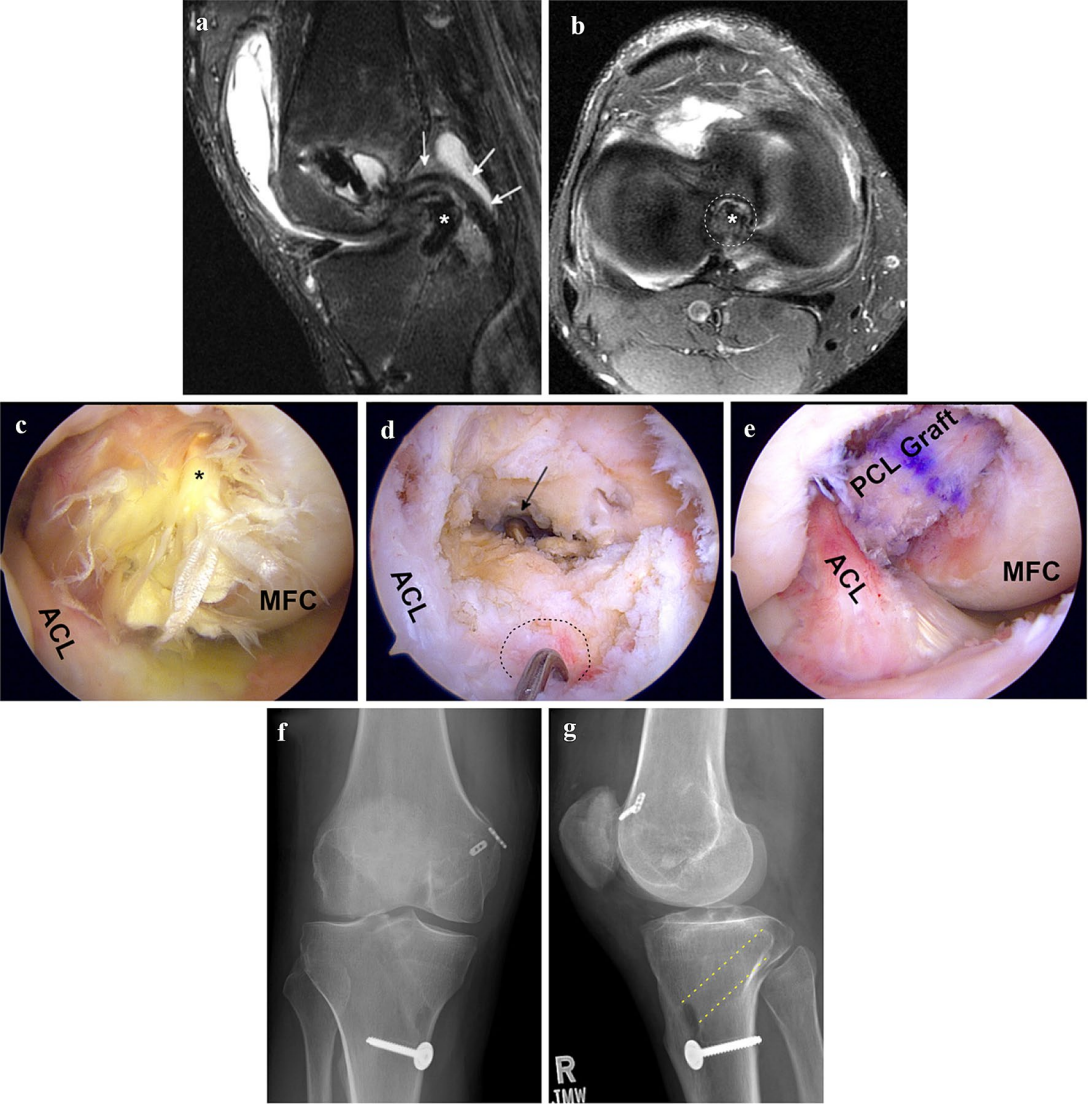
Posterior cruciate ligament graft failure. Patient with atraumatic PCL graft failure of the right knee. T2-weighted sagittal (a) and axial (b) MR images showing PCL graft failure and misplaced tibial tunnel (too anterior and too proximal). Note scarring of remnant PCL fibers imitating PCL continuity (white arrows). Arthroscopic images demonstrating graft failure (c), misplaced tibial tunnel (d), and revision PCL graft (e). Postoperative anterior–posterior (f) and lateral (g) radiographs demonstrating new anatomic tibial tunnel. white/black dashed lines, misplaced tibial tunnel; yellow dashed lines, new anatomic tibial tunnel; black arrow, new anatomic tibial tunnel during revision PCL reconstruction; *deficient PCL graft, *ACL* anterior cruciate ligament, *MFC* medial femoral condyle, *MR* magnetic resonance, *PCL* posterior cruciate ligament
